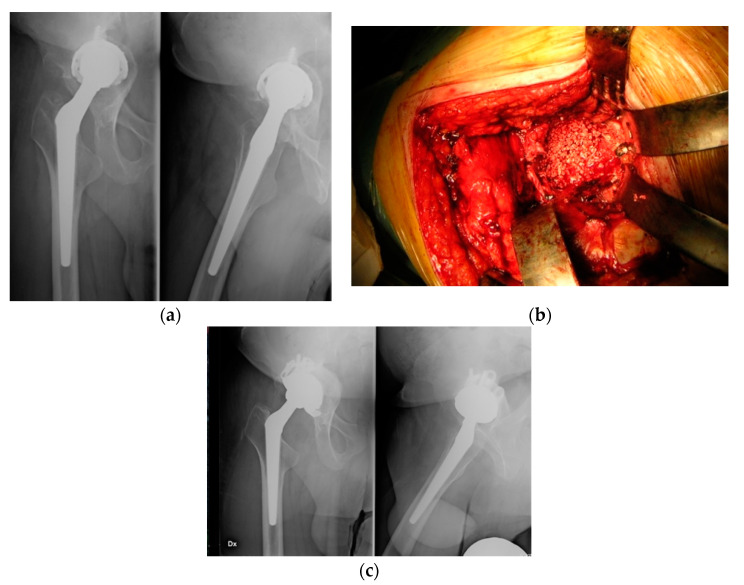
Clinic case: 76 y female with loosening of the acetabular cup (a) Pre-operative x-rays, (b) Intraoperative pictures show the acetabulum impacted with Engipore chips filling the bone defect (Paprosky 3a), (c) Post-operative x-rays at the last follow-up (3.2 years) showing good osteointegration of the implant.
